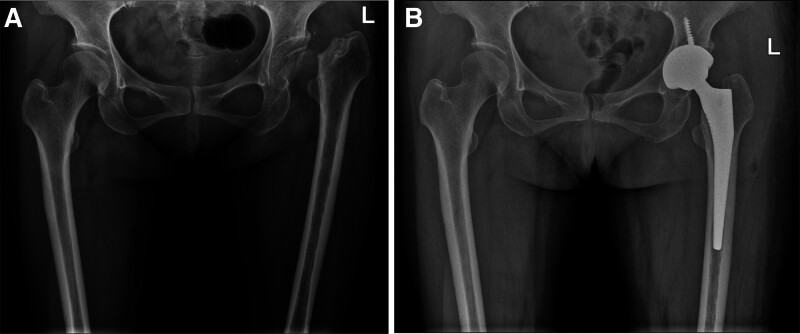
(A–B) Radiographic progression of the left hip: (A) Preoperative anteroposterior projection radiograph demonstrating disappearance of femoral head dissolution. (B) Postoperative anteroposterior projection radiograph demonstrating the artificial prosthesis was in a good position without loosening or fracture.
